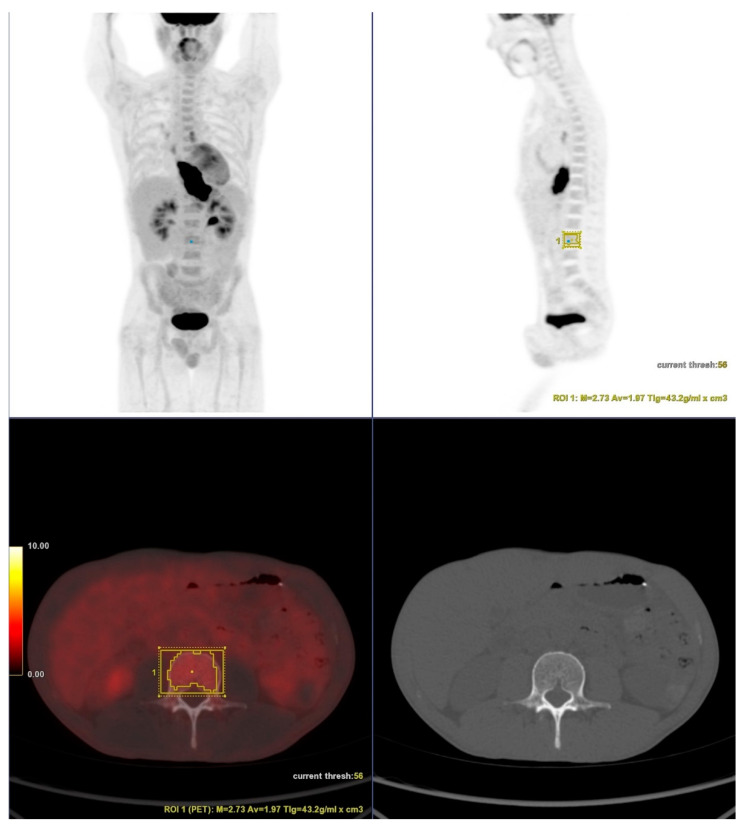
18F-FDG PET/CT showing a patient with esophageal cancer. Volumes of interest (VOIs) were drawn over in one of the L3-L5 vertebral bodies, preferably L3. Care was taken to exclude bones with fractures, as shown in CT slice. Automatic isocontour was generated, and the mean SUV was calculated.
